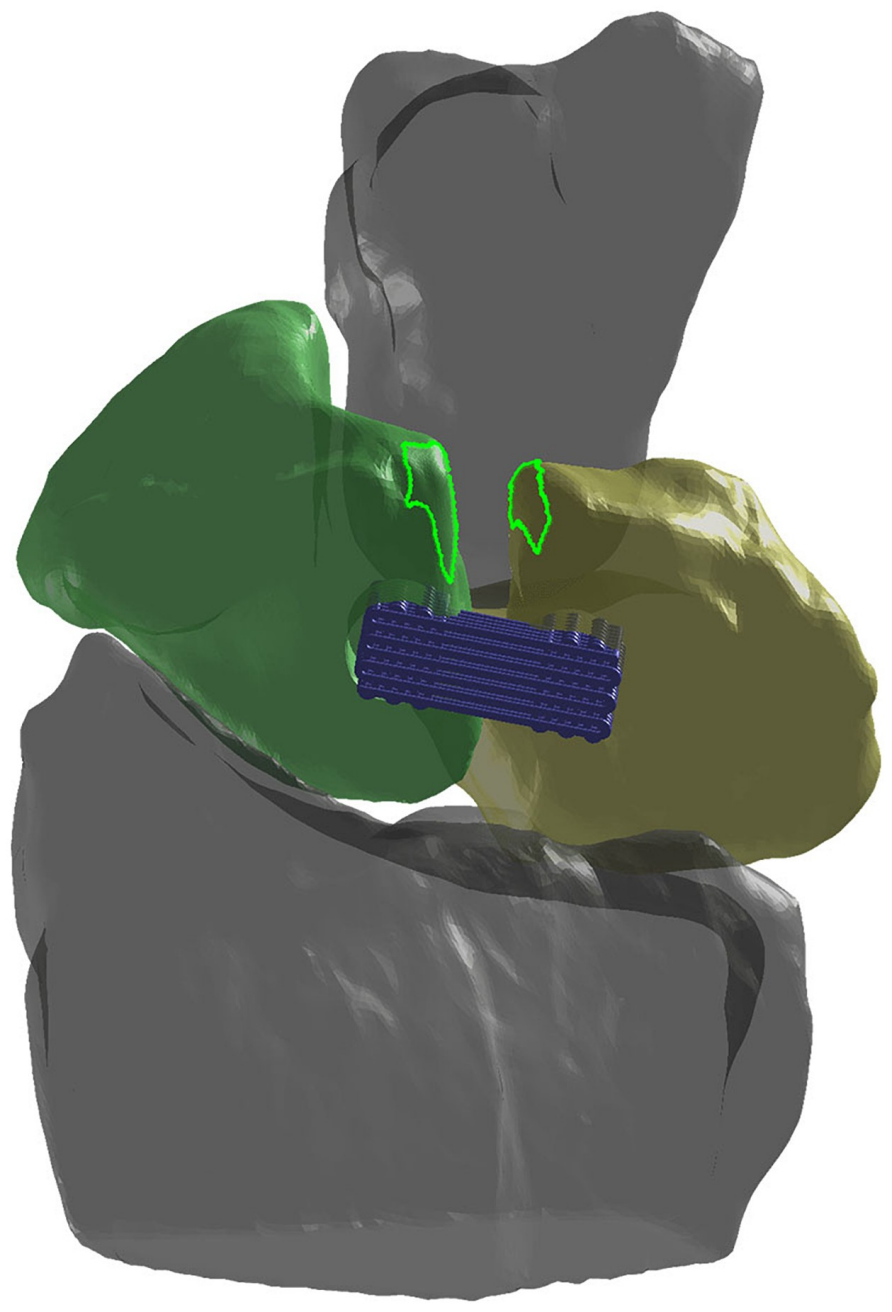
The 3D anatomical model created from computed tomography scan. The model consists of capitate (grey), scaphoid (green), lunate (yellow), and radius (grey) bones. Bone positions in dorsal view of anatomical model correspond to maximum wrist flexion. The attachment sites of the dorsal SLIL are depicted with green dotted lines on both scaphoid and lunate. The dorsal SLIL attachment sites are derived from MRI scan of intact native SLIL with the wrist at neutral position and projected onto the anatomical model created from computed tomography scan. The BLB scaffold is placed at a position proximal to the native attachment sites.
